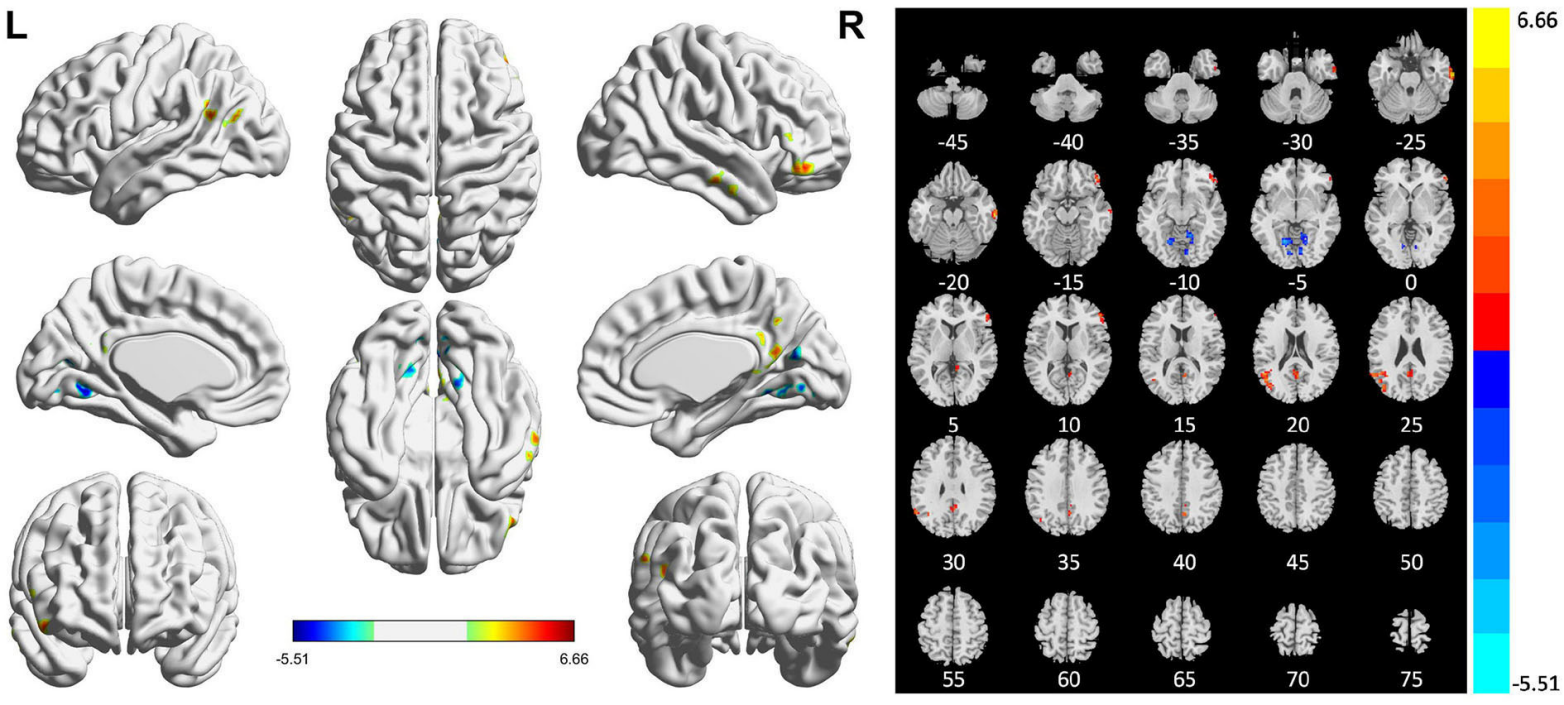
Statistically significant differences between post-stroke insomnia (PSI) patients and healthy controls are shown in the amplitude of low-frequency fluctuation (ALFF) map of the whole brain with magnetic resonance imaging (MRI). Patients with PSI showed a significant ALFF increase in the bilateral middle temporal gyrus, right inferior temporal gyrus, right inferior frontal gyrus, right limbic lobe, right precuneus, left posterior cingulate gyrus, and left middle occipital gyrus (warm colors), as well as a decrease in the bilateral lingual gyrus with decreased ALFF values (cold colors). A T-score bar is shown below and right. Red and blue denote increases and decreases in ALFF, respectively.
